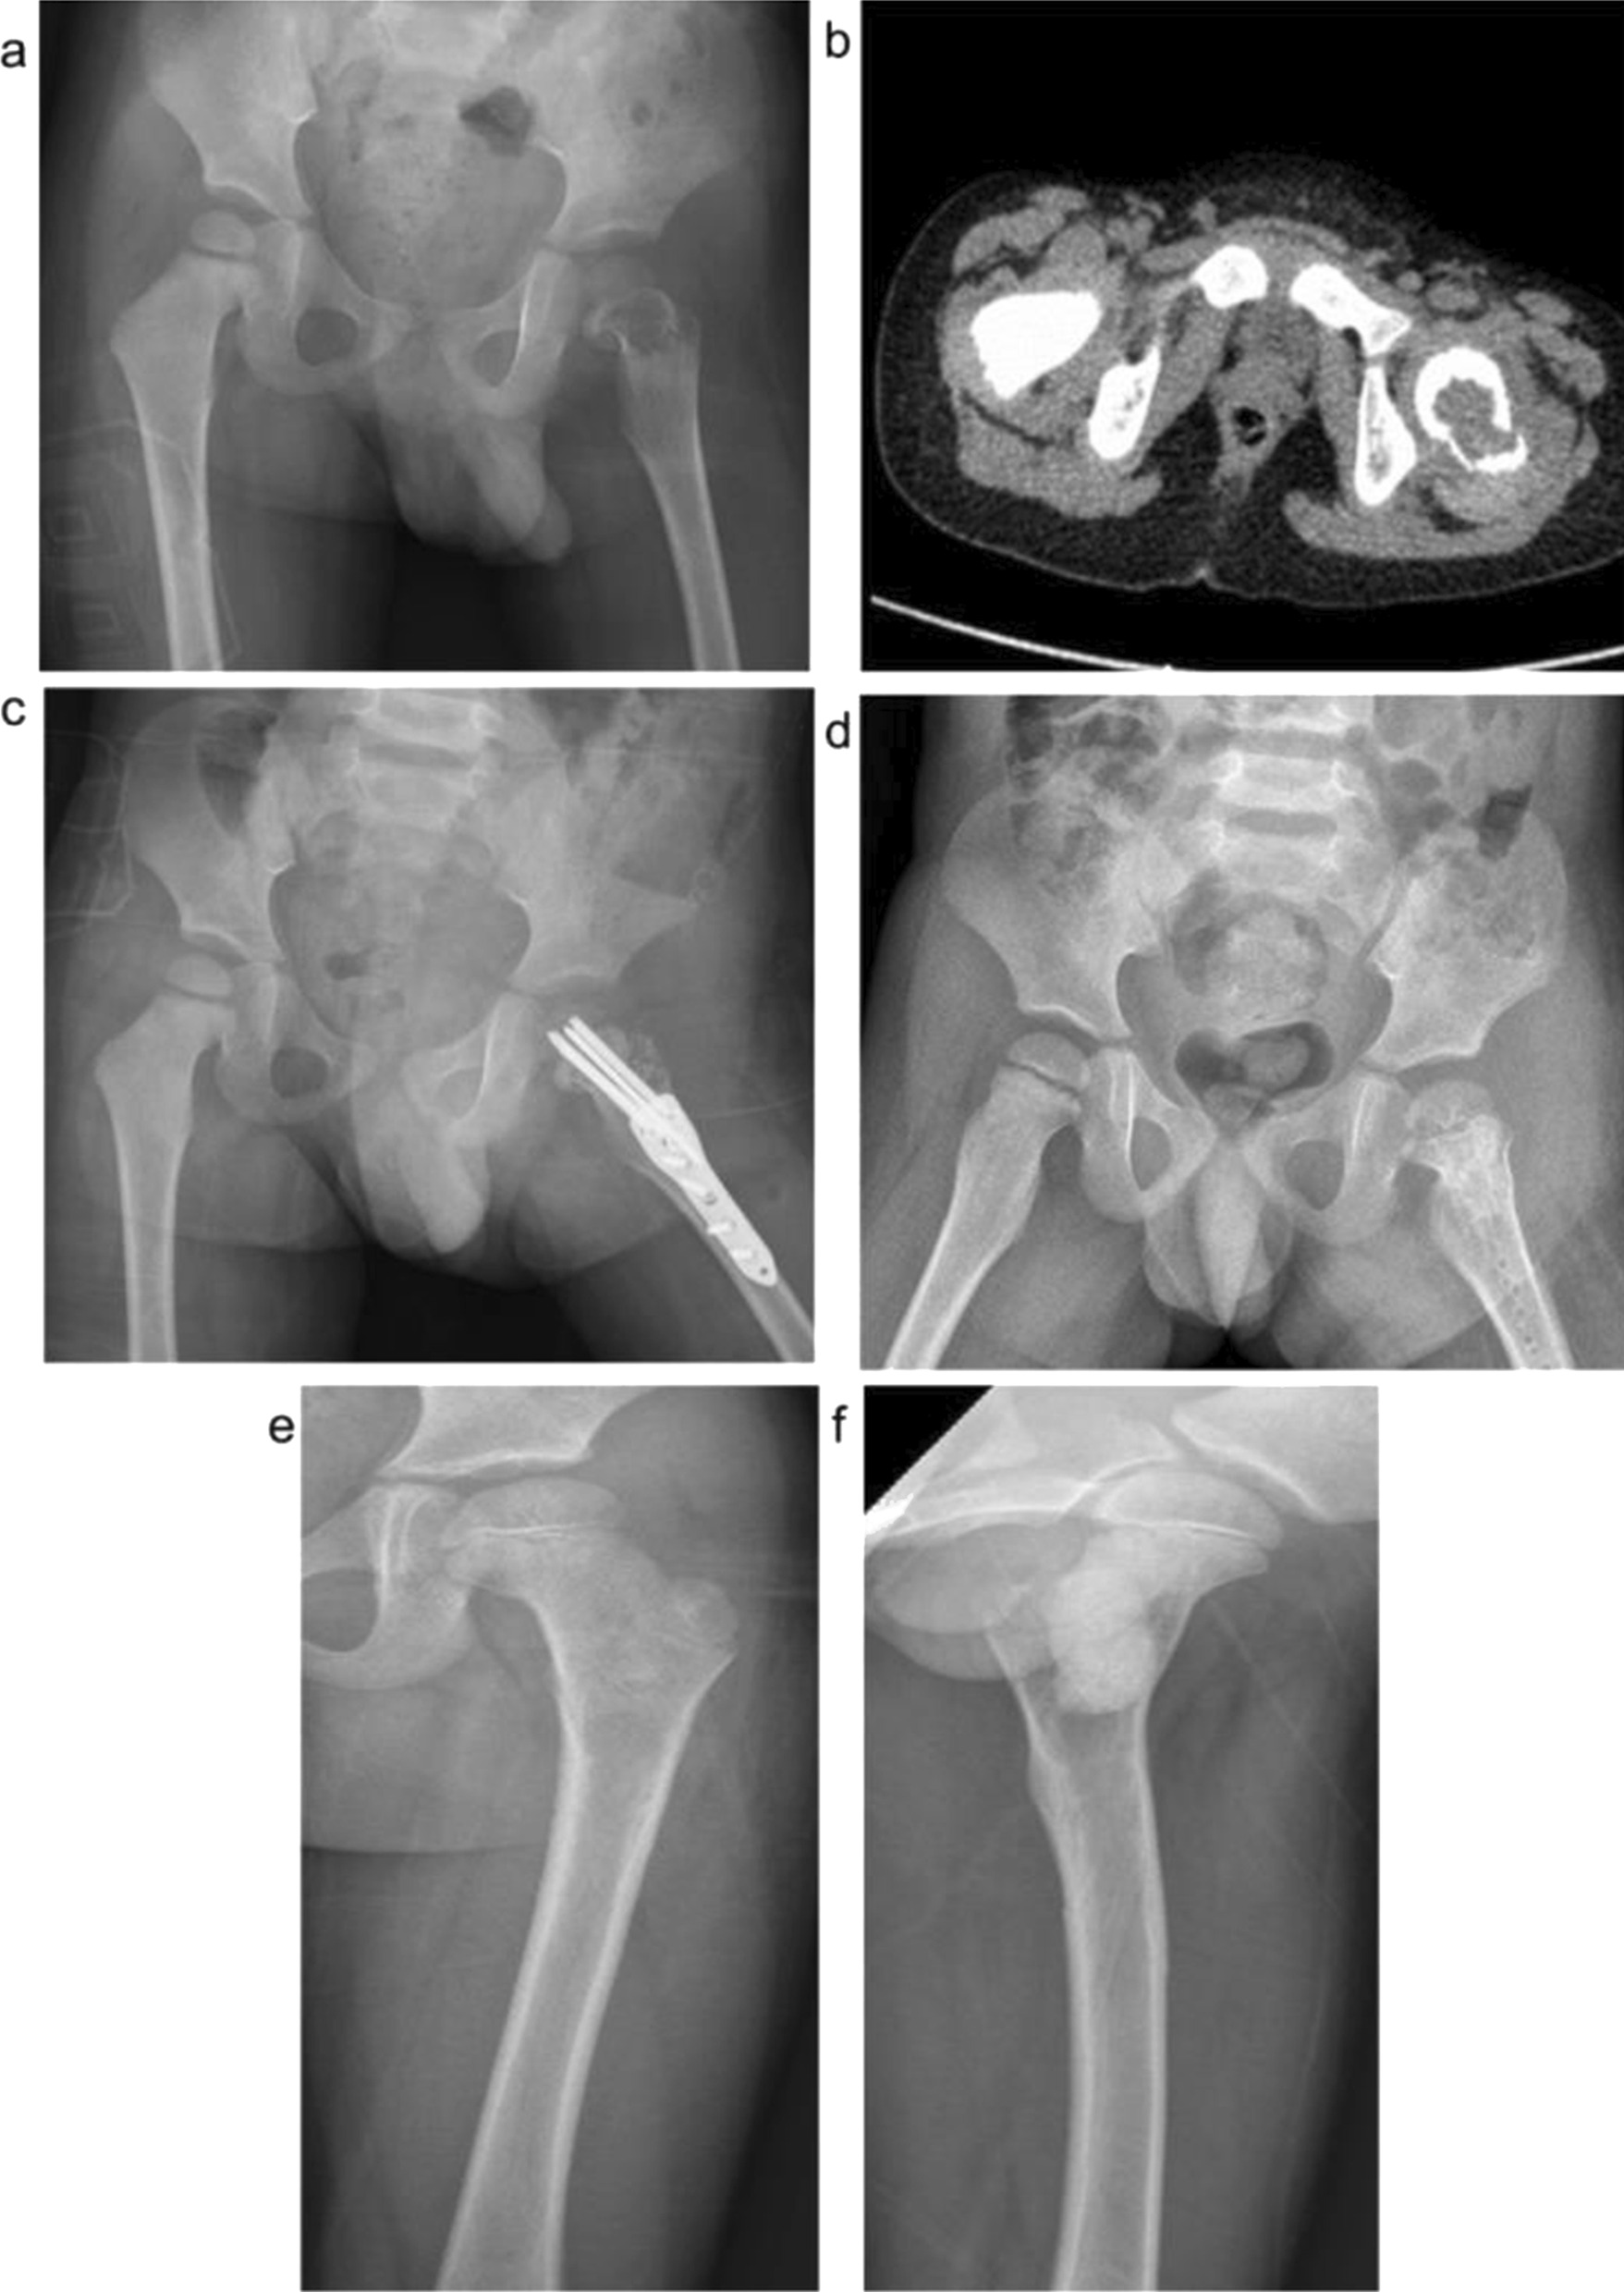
A 3-year-old girl diagnosed with Langerhans cell histiocytosis of the left femoral head and neck with a pathological fracture. Surgical treatment of the left femur tumor was performed by curettage and artificial bone + autogenous iliac bone grafting. The pathological fracture was treated by orthopedic reduction and internal fixation with the Locking Compression Pediatric Hip Plate™. a. Preoperative X-ray; b. Preoperative CT; c. Postoperative; d. 6 months after operation; e. 4 years after operation, positive X-ray; and f. 4 years after operation, lateral X-ray
